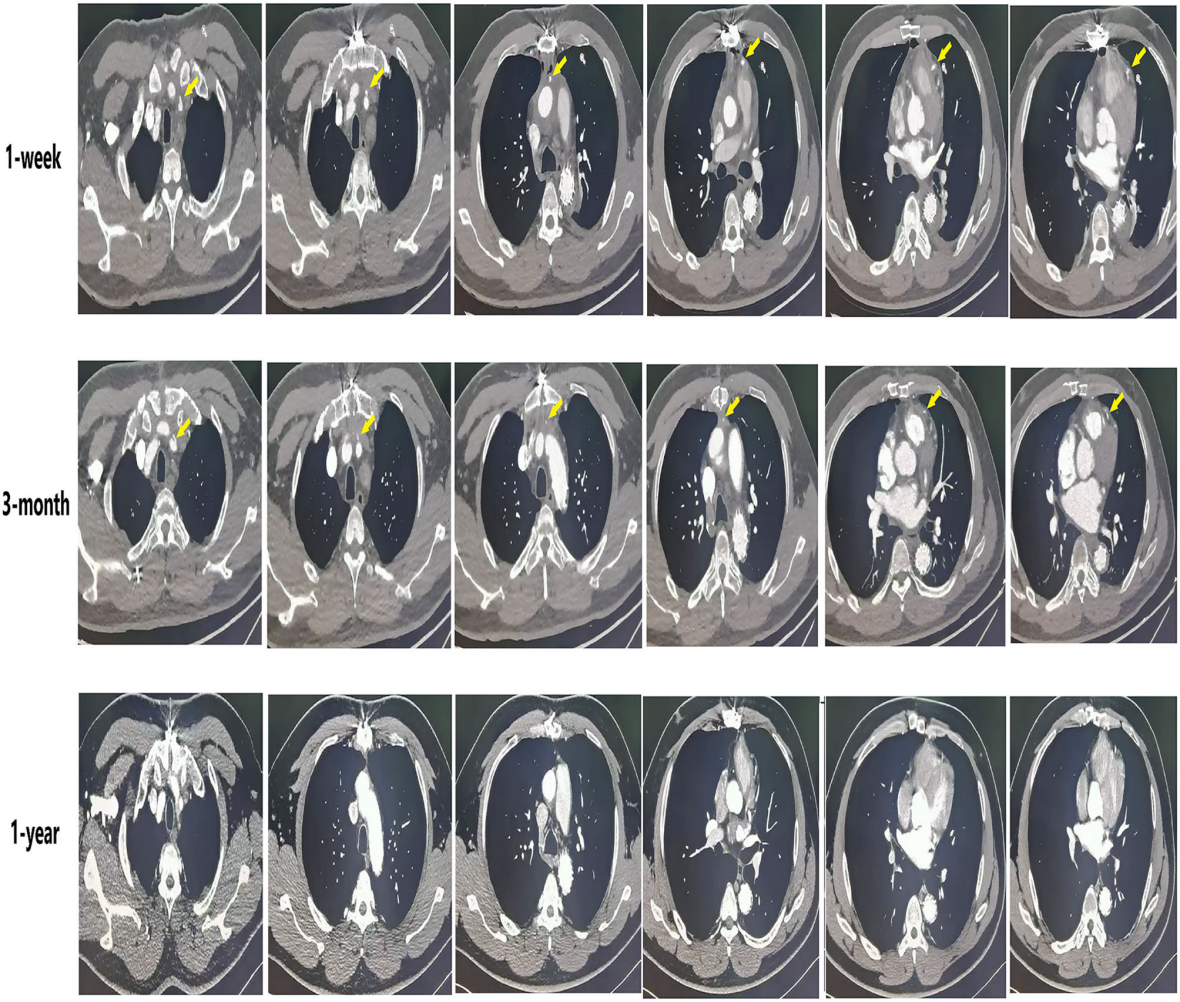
The condition of SVG-non-AO grafts at different times (coronary CT angiography).
